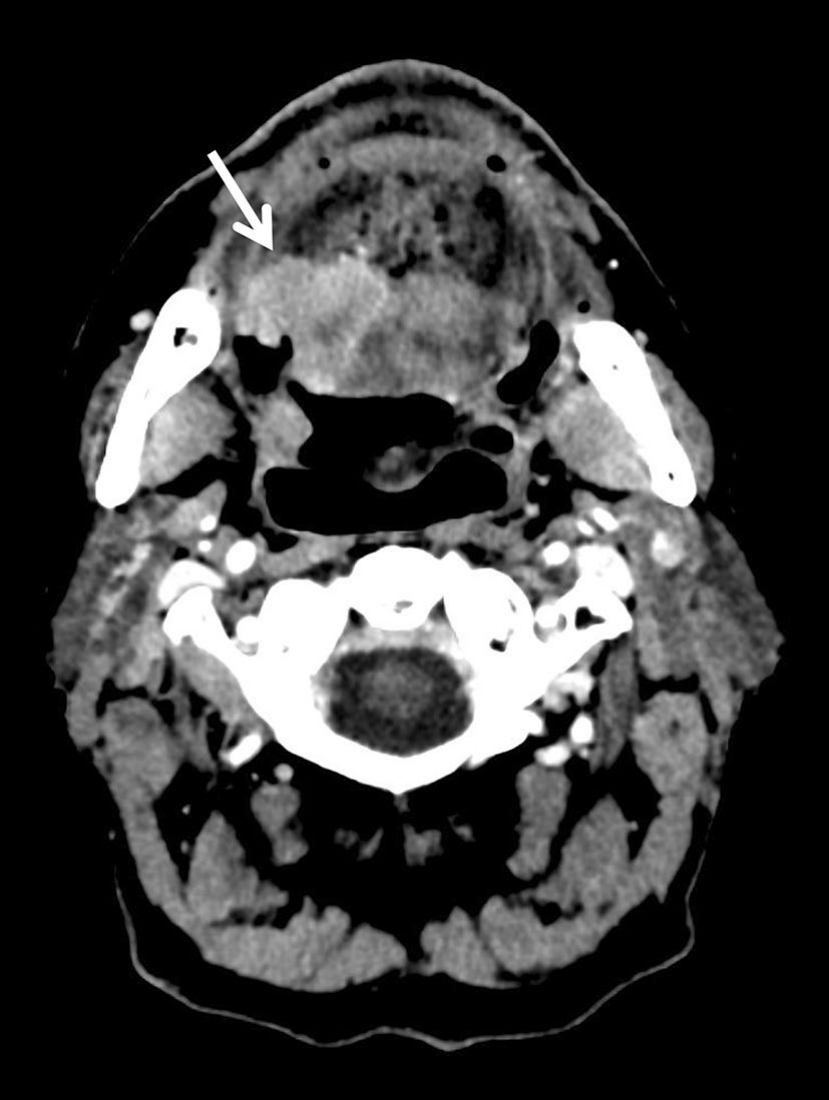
Axial CT slice showing a necrotic ulcerated (arrow) p16− tumour of the base of the tongue in a 59-year-old male former smoker.
